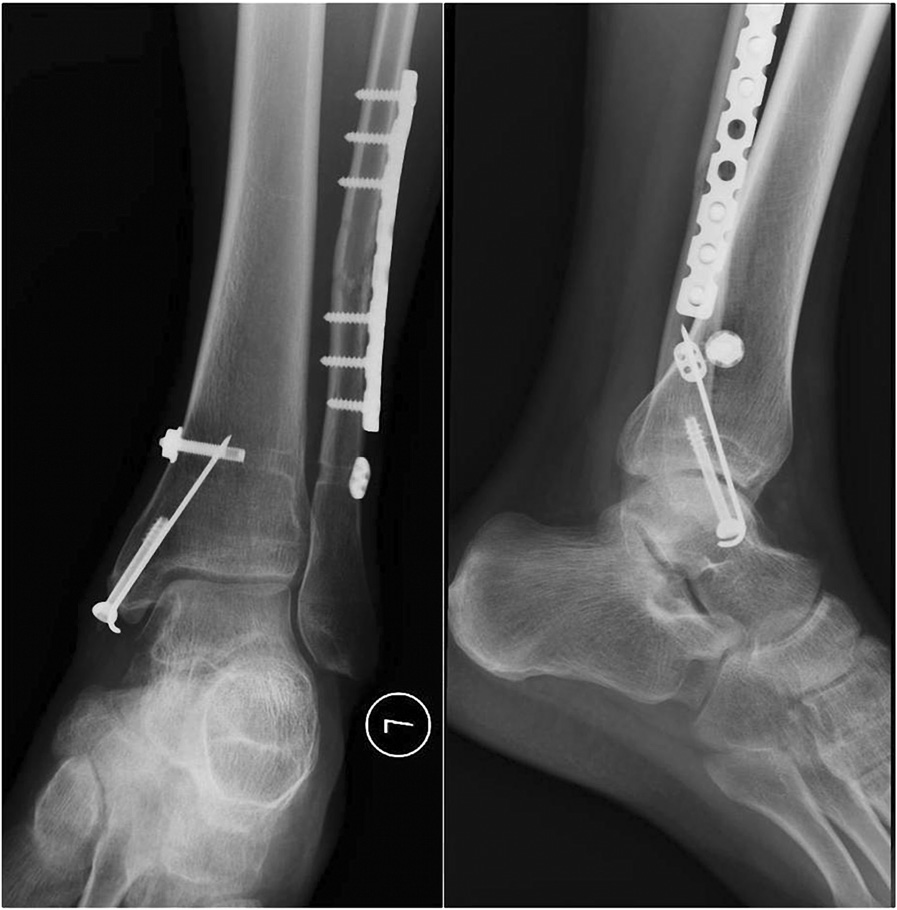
Mortise and lateral view of the ankle joint radiograph showed union of the fibular and medial malleolus fractures and a normal syndesmosis at 1 year follow-up after operation.
